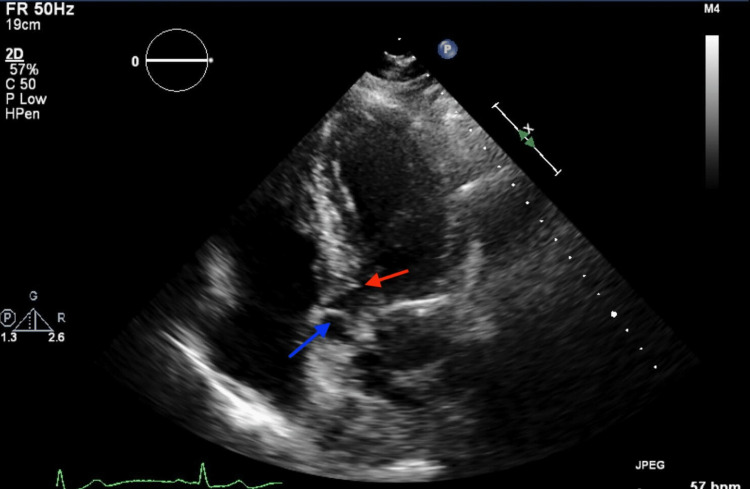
Transthoracic echocardiogram (apical 5 chamber view) showing subaortic membrane (red arrow). Aortic valve leaflets are indicated by the blue arrow.
